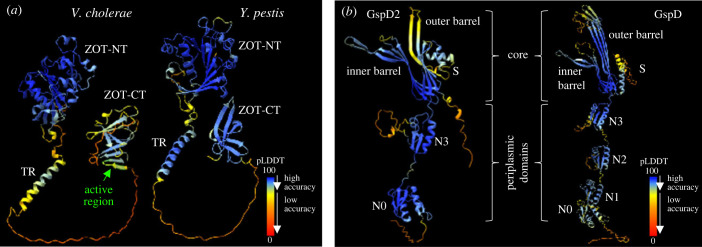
YpfΦ-encoded molecules exhibit homology to Zot—a virulence factor of *V. cholerae* (*a*) and secretin of the T2SS (*b*). Predicted structures are coloured based on the pLDDT confidence measure. (*a*) Predicted three-dimensional protein structure of *Y. pestis*'s Zot reveals a partial structural similarity to Zot of *V. cholerae*. Both proteins have a transmembrane region (TR) as well as C-terminal (ZOT-CT) and N-terminal (ZOT-NT) domains. The *Y. pestis’* Zot lacks the active region (FCIGRL) present in *V. cholerae*. (*b*) Predicted three-dimensional protein structures reveal differences in the protein core as well as in the periplasmic domain that is relevant for substrate specificity. The main domains (Nx and S) are labelled. The nomenclature is based on the resolved structure of the GspD of *Klebsiella pneumoniae* [31].
